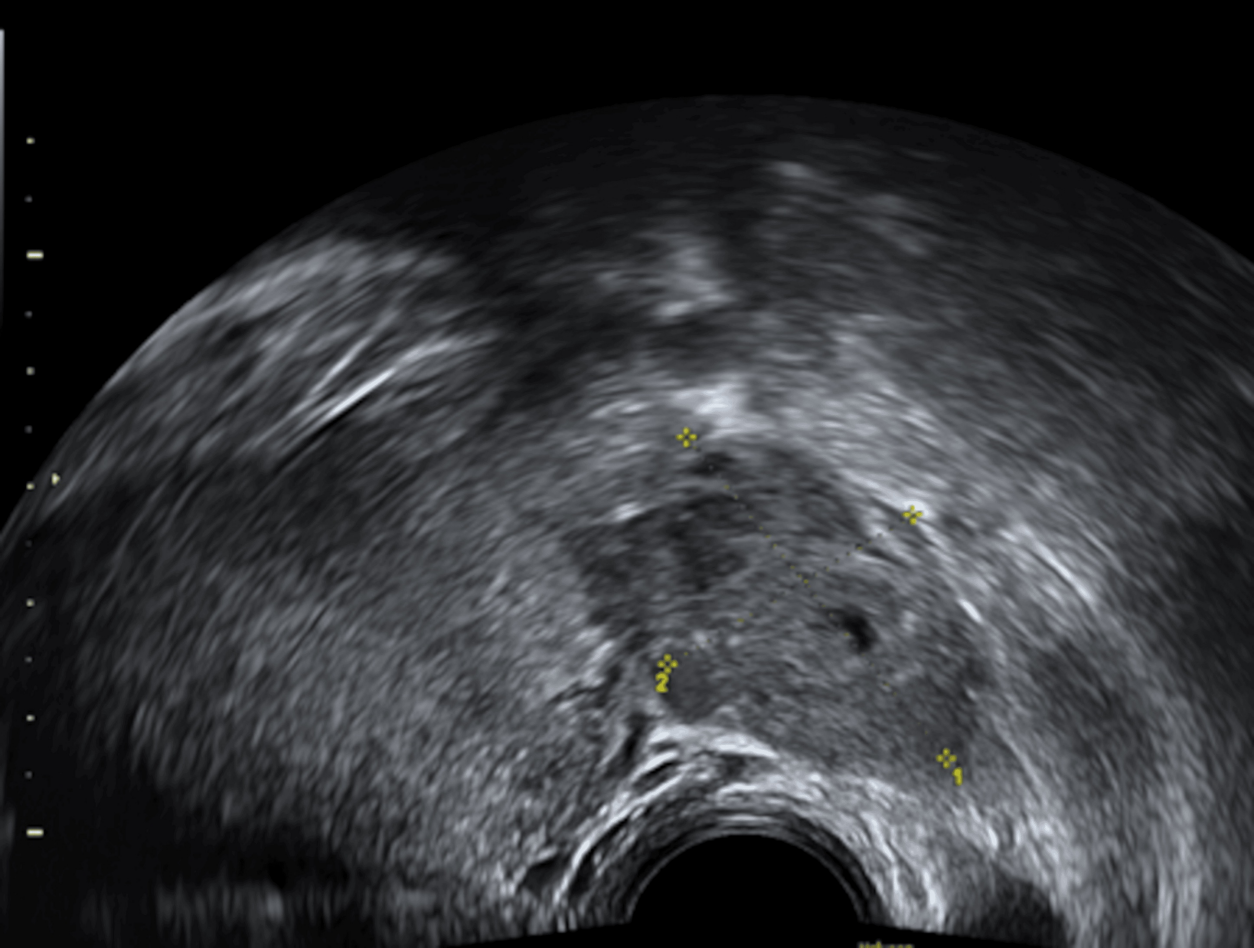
Ultrasound images suggesting ectopic pregnancy with a right ovarian mass
